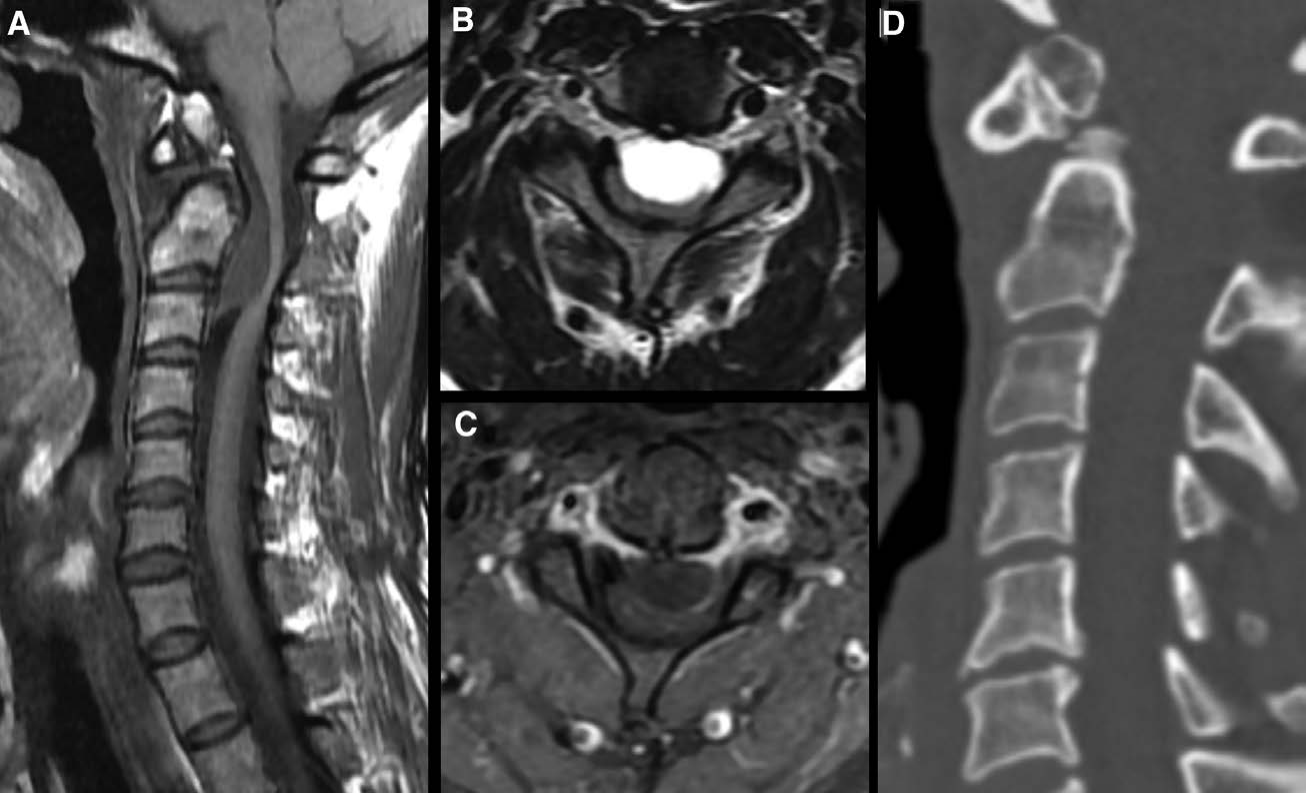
(Case 2) Preoperative spine magnetic resonance imaging (MRI) reveals a 30 mm × 15 mm mass at the level of C2–C3. The lesions have (A) an isointense to low-intensity signal on T1-weighted MRI, (B) high-intensity signal on T2-weighted MRI, (C) no enhancement after contrast-enhanced MRI, and (D) computed tomography sagittal slices show a circumferential corticated mass consistent with OS odontoideum.
